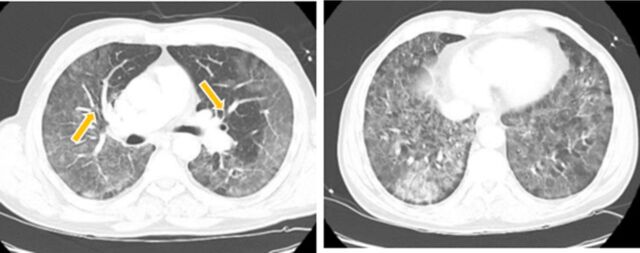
Computed tomography images show filling defect (arrows, left panel) suggestive of pulmonary emboli and extensive ground-glass opacities (right panel) in bilateral lungs.
